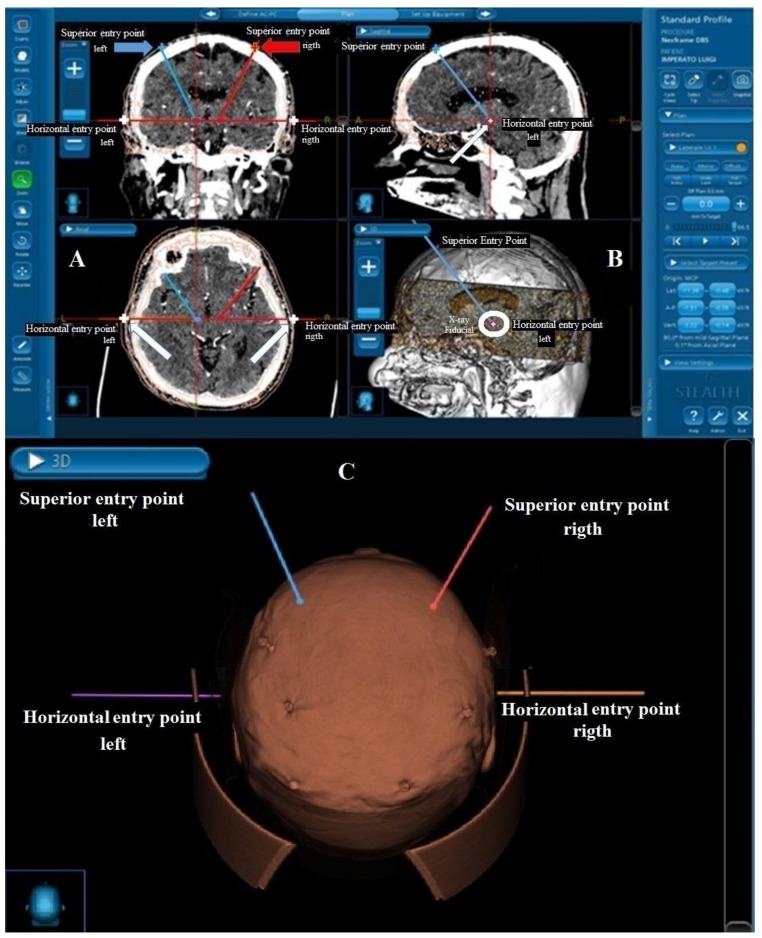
(A) Coronal (upper) and axial (lower) CT scan slides. The trajectories and superior entry points (red/blue arrows and red/blue cross) planned using the Medtronic Stealth-Station Framelink software package are reported. The inclined blue line represents the trajectory targeting the left STN, while the inclined red line represents the trajectory for the right STN. The horizontal red line crossing the target points from right to left is the Z plane. When this line crosses the skin (white arrows and cross) it is easy with the neuronavigational system to establish the horizontal entry point corresponding to the target point; (B) That which is reported in (A) is here represented in sagittal view. The projection of the horizontal entry point is represented by the red dot (white arrows and white cross inside the red dot). The inclined blue line represents the trajectory of the electrodes (upper slide); in (B), lower slide, a X-ray visible fiducial (white circle) is reported. (C) The 3D representation of the surgical planning and fiducial screws.
